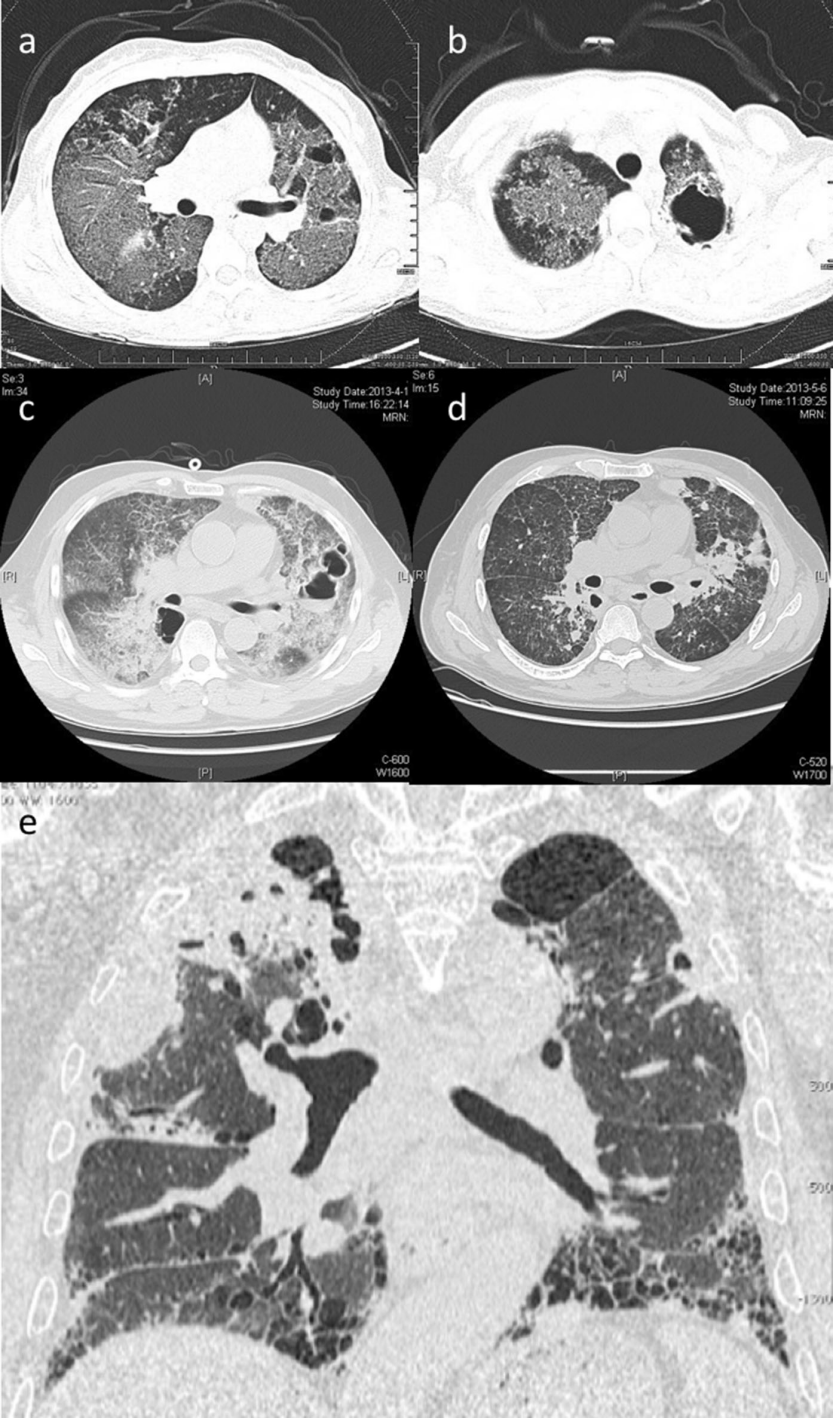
Lower respiratory tract manifestations of talaromycosis. a & b: CT Chest demonstrating extensive bilateral ground glass changes and multiple bullae, in a 34-year-old immunocompromised female with a *STAT3* mutation [59]. c: CT Chest with diffuse interstitial infiltrates and multiple cavitary lesions in a 57-year-old man following steroid use [60]. CT Chest d following treatment, demonstrating marked regression of interstitial and cavitary lung disease. e CT Chest demonstrating right upper lobe infiltration with Tm, bibasilar subpleural reticular changes, traction bronchiectasis and honeycombing in a 71-year-old, immunocompetent patient with idiopathic pulmonary fibrosis [61]
